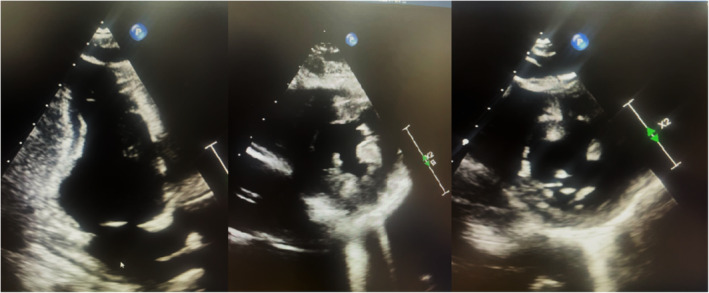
An echocardiograph of the patient shows inferior and basal segment hypokinesia and an Ejection Fraction of 50%.
